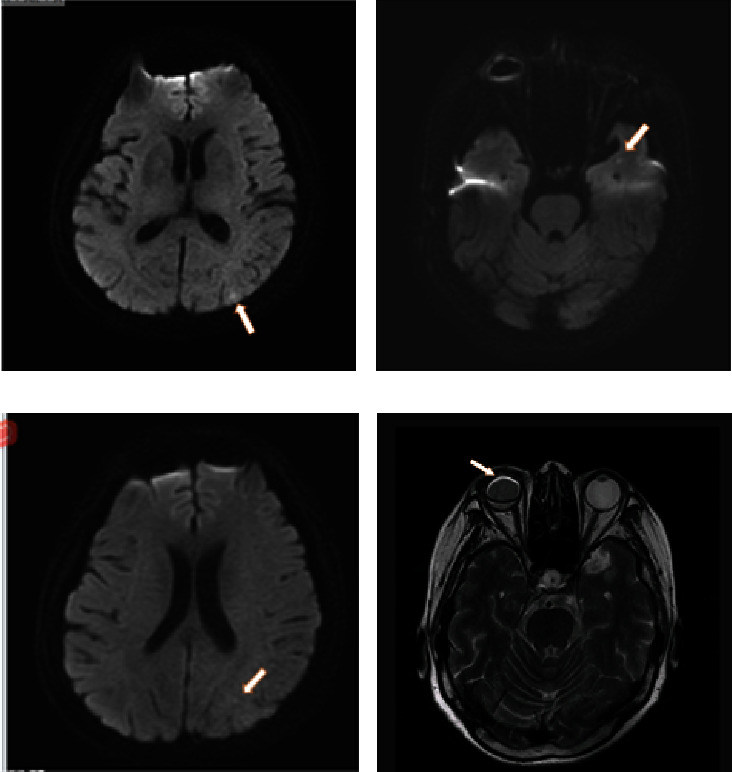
Head/eye MRI on day 18 of admission. On the 18th day of admission, head and eye MRIs were performed. As the arrows indicated, DWI showed slightly high signal intensity in the left occipital lobe (a), left temporal lobe (b), and parietal lobe (c). As the arrow indicated, the signal of the right eyeball was not uniform, and the vitreous body displayed an oval slightly long T1 and T2 signal shadow (d).
